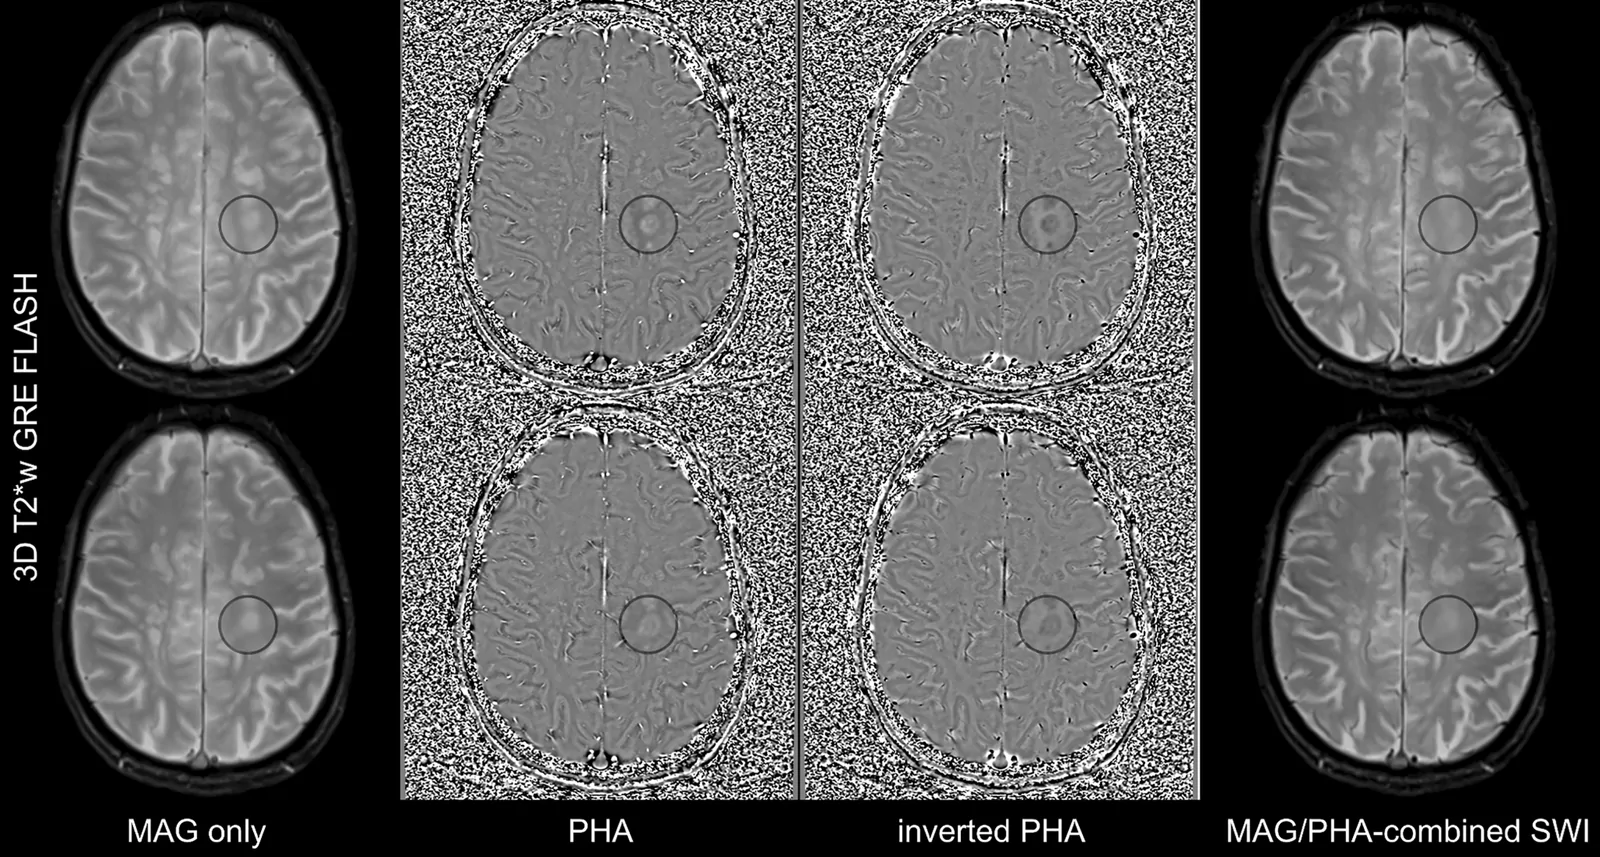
Detection of paramagnetic rim lesions (*PRLs*) as a new diagnostic and potentially prognostic MRI biomarker for MS by the processed phase component (*PHA*) of susceptibility-weighted imaging (*SWI*). PRL (black circled), meeting the required identification/exclusion criteria (Table 5) and confirmed on two consecutive slices by high-resolution 3D T2*w GRE FLASH (0.51 × 0.51 × 2.67 mm^3^) in a patient with proven MS. Note that T2-hyperintensity of MS lesions in T2*w magnitude (*MAG*) only reconstructions may null PRL-hypointensity in MAG/PHA-combined SWI [58]. Therefore, PRLs should be evaluated primarily on PHA (Table 6). White matter surrounding the PRL appears darkened on PHA (and brightened on inverted PHA), which is simply a Mach effect in the magnifier circle.
